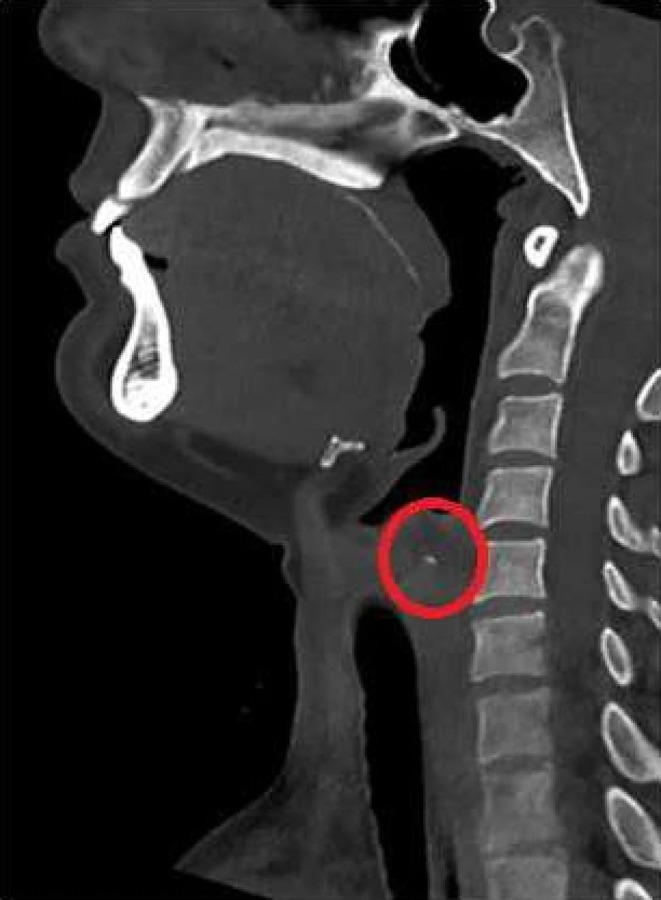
Computed tomographic sagittal view demonstrating contrast medium accumulation in left piriform sinus
